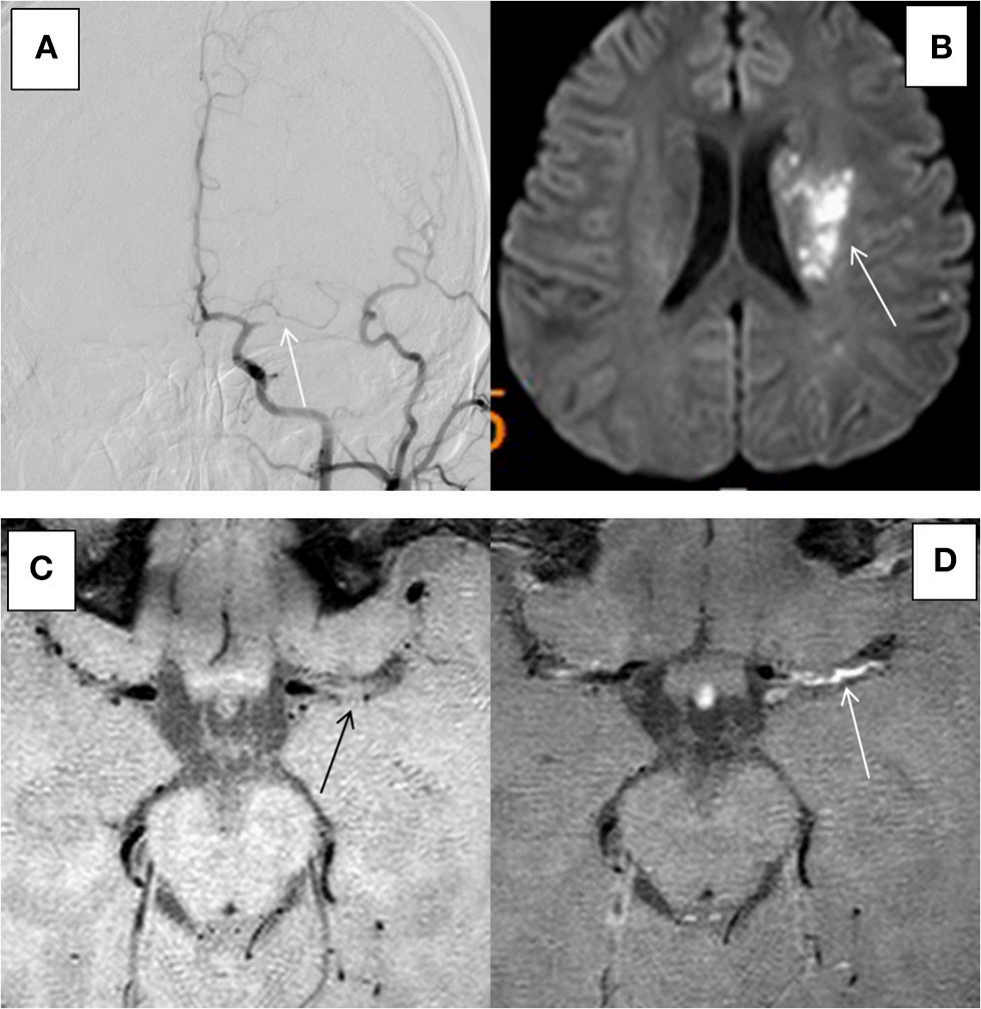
HR-MRI of a culprit MCA occlusion in an 28-years-old male with a history of previous hypertension. (A) DSA showed occlusion of the left MCA. (B) DWI showed that the left side of the paraventricular was limited by diffusion. (C) HR-MRI showed plaque formation in the Ml segment of MCA. (D) High-resolution enhanced Tl WI showed marked enhancement of the Ml segment of MCA.
